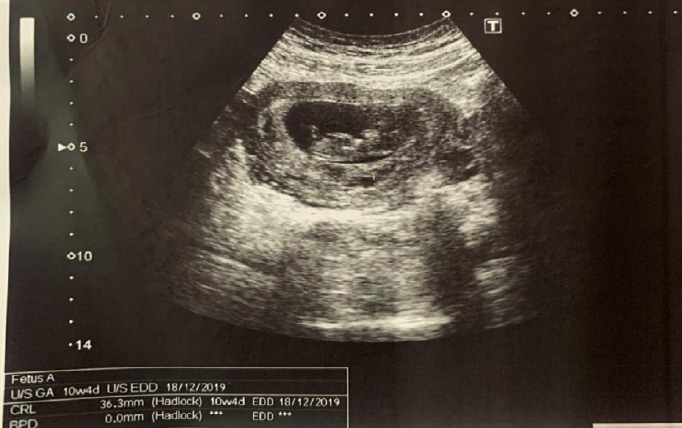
Transabdominal ultrasonography showed a single live intrauterine pregnancy of 10 weeks and 4 days with CRL measuring 36.3 mm.
